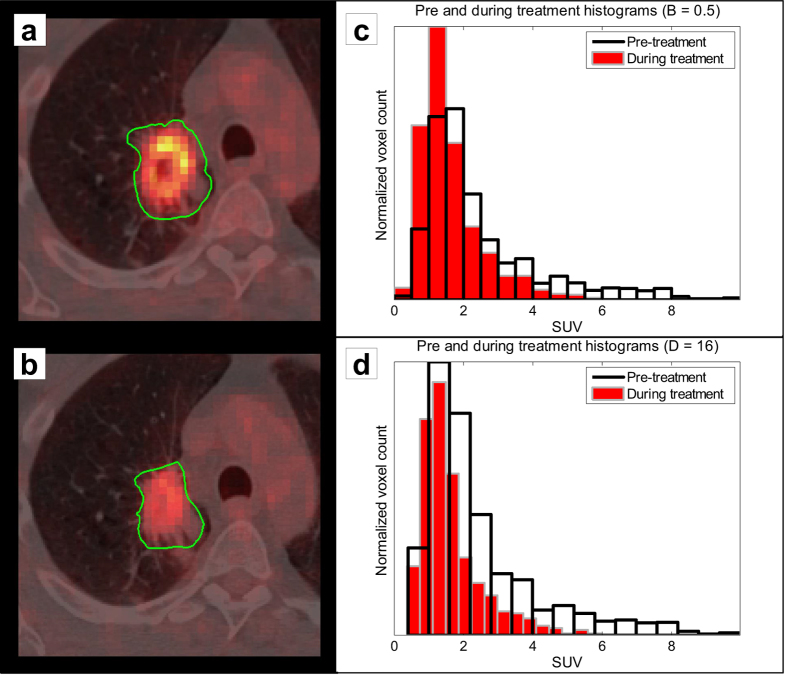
Left column:* Representative images of sequential imaging for one patient, showing pre-treatment imaging (a) and imaging during the second week of radiotherapy (b). The tumor delineation is outlined in green. Both images are displayed with the same window/level settings. *Right column:* Histograms of the pre-treatment and during treatment images, resampled with a fixed bin size (i.e. intensity resolution) (c) or a predefined number of bins (d). In (d), one can appreciate the difference in resulting intensity resolution when resampling with a fixed number of bins. Pre-treatment and during treatment intensity resolutions were 0.6 and 0.37 [SUV], respectively
